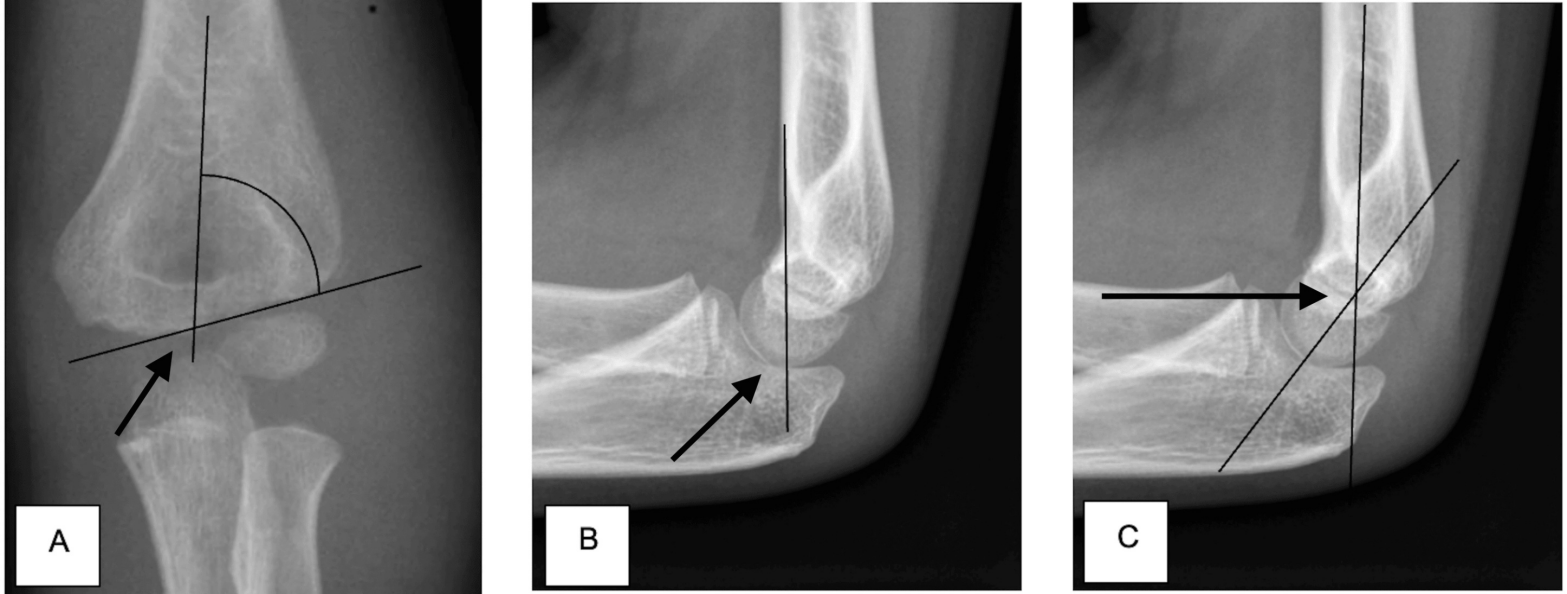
Parameters for judging loss of fracture reduction (A) Bauman’s angle (BA) - this angle is formed by the humeral axis and capitellar physis on the AP radiograph. (B) Anterior humeral line (AHL) - a line drawn along the anterior border of the distal humerus in the proximal fragment on the lateral radiograph; the line usually intersects the capitellum. (C) Humerocapitellar angle (HCA) - the angular relationship between the humeral shaft and capitellum on the lateral radiograph.
